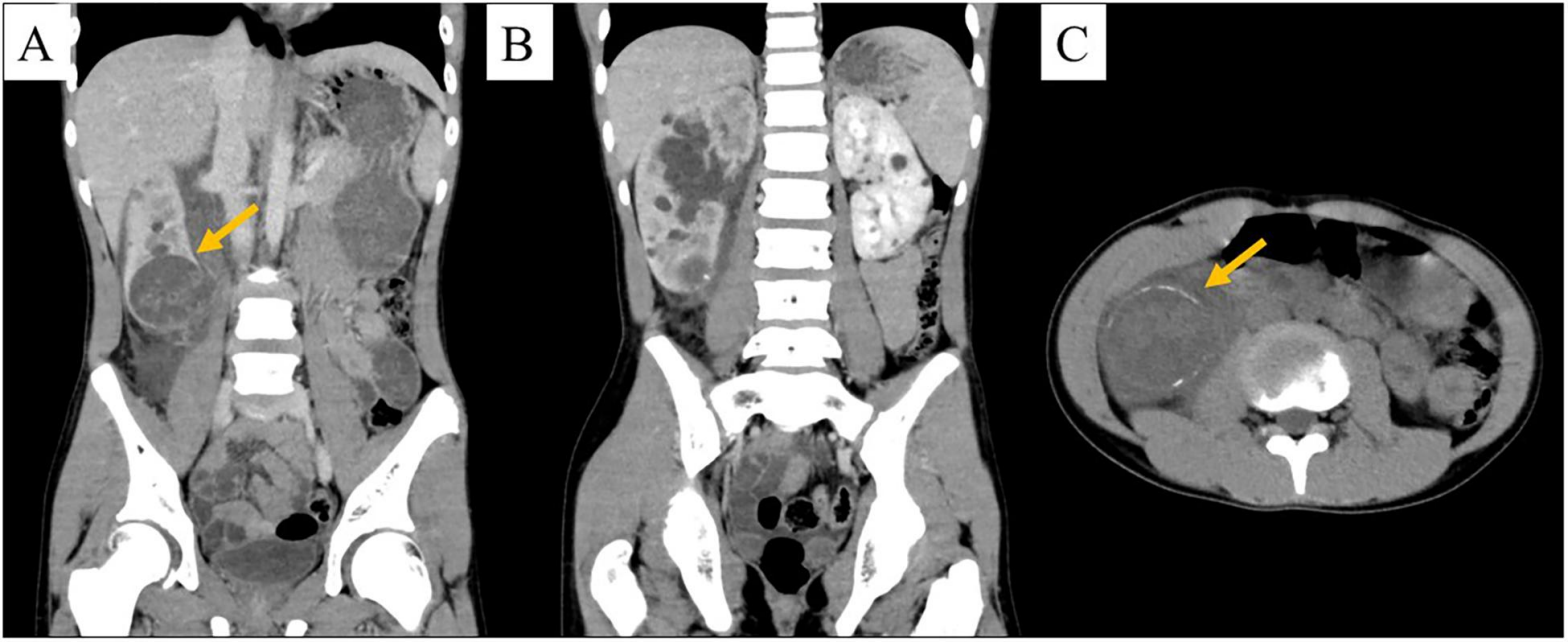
Abdominal computed tomography. (A,B) Contrast-enhanced. (C) unenhanced. (A) Heterogeneous ∼5-cm cystic lesion at the inferior pole of the right kidney (orange arrow) with focal wall discontinuity compatible with a rupture. (B) Numerous bilateral renal cysts, with slightly reduced enhancement of the right kidney compared with the left kidney (etiology undetermined). (C) The same cystic lesion with peripheral rim calcifications (orange arrow).
